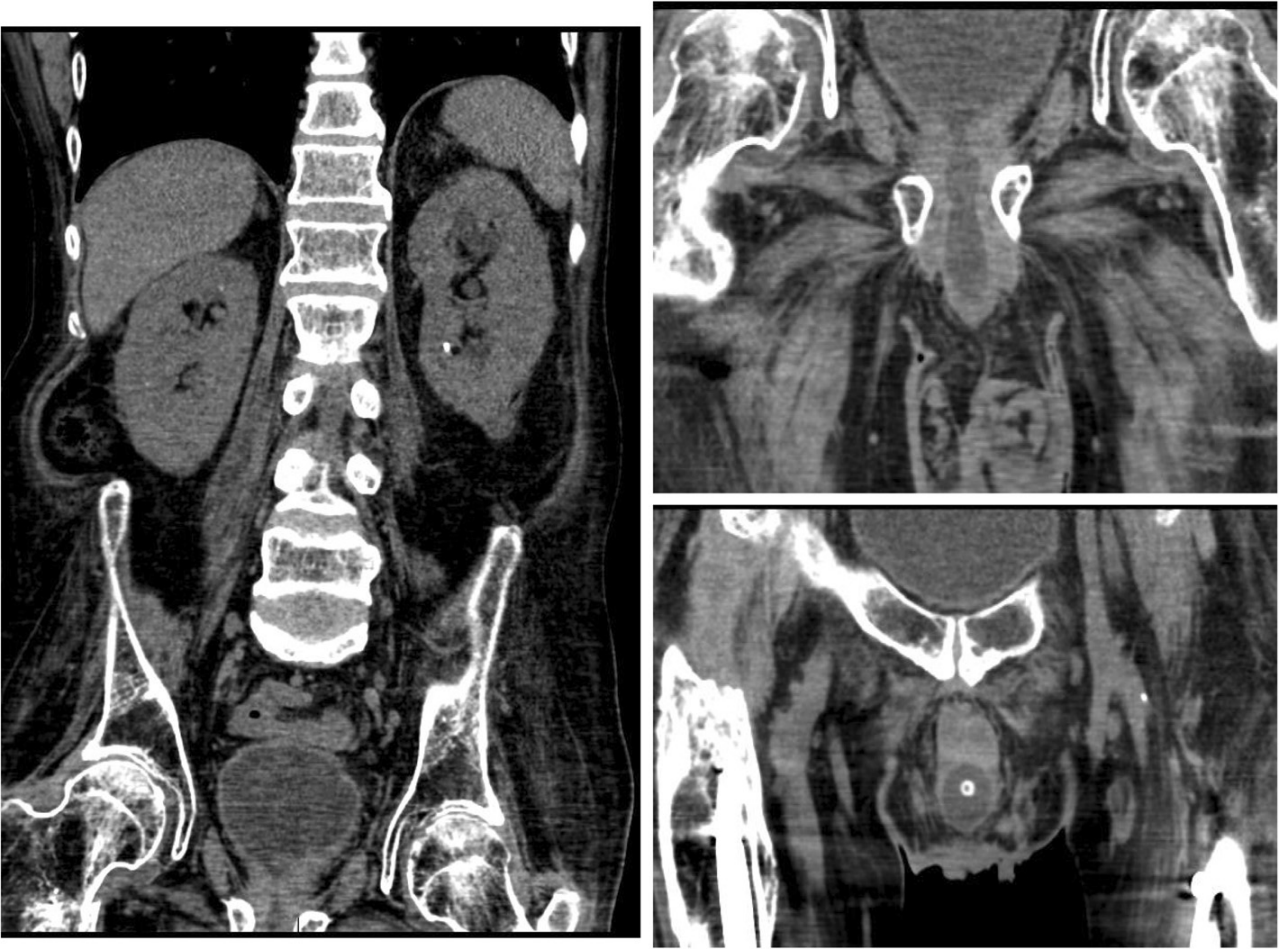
Case 1. Left panel: Coronal section of non-contrast CT of abdomen revealed full bladder; Foley catheter was not seen within the urinary bladder. Right Top panel: Coronal section of CT of pelvis showed fluid distension of the membranous and prostatic urethra. Foley catheter was not seen in bladder or in proximal urethra. Right bottom panel: Coronal section of CT of pelvis revealed balloon of Foley catheter in the proximal penile urethra
